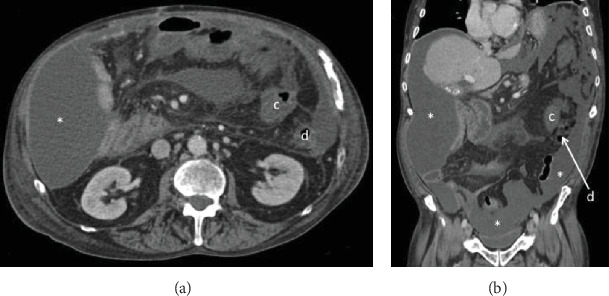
Abdominal computed tomography after Ladd's procedure in (a) transverse and (b) coronal planes demonstrating intraabdominal loculated ascites (∗) in Morrison's pouch, right and left paracolic gutters, and pelvis. There is resolution of small-bowel obstruction after Ladd's procedure with the cecum (c) positioned in the left upper quadrant medial to the descending colon (d).
